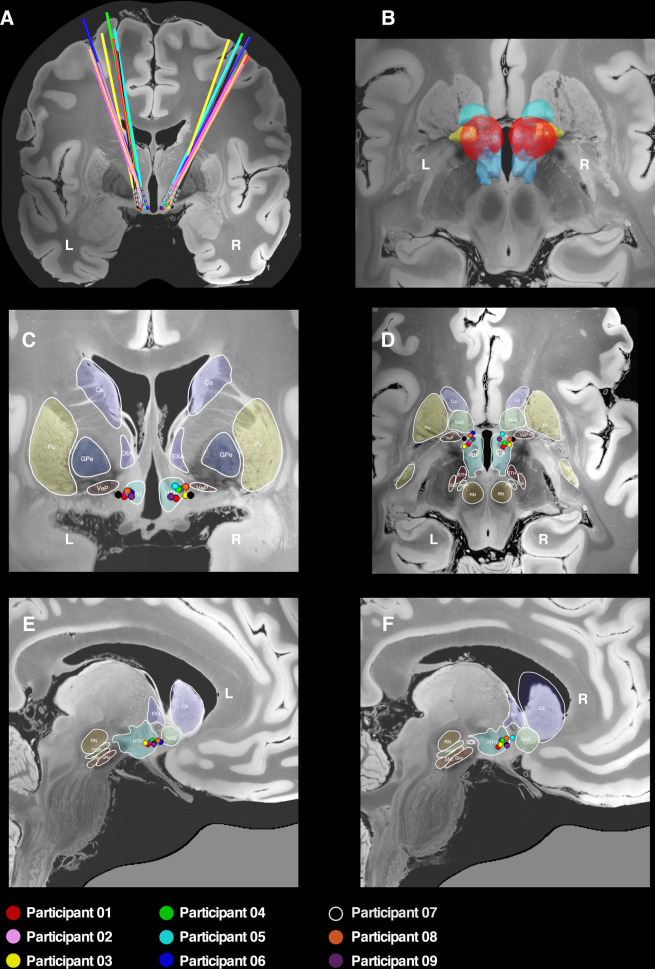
Localisation of electrodes and active contacts. DBS electrodes were localised with the Lead-DBS toolbox and represented in common ICBM 2009b nonlinear asymmetric space incorporating a 7-Tesla MRI at 100 micron resolution^73^ with subcortical parcellations derived from a recent high-resolution atlas^74^. A Three-dimensional reconstruction in the coronal plane showing electrode trajectories for the nine participants. B Three-dimensional reconstruction in the axial plane showing the distribution of the aggregated stimulation field across the cohort (red), which can be seen to encompass the posterior segment of the nucleus accumbens (light green), the ventral pallidum (yellow) and the hypothalamus (blue). C Two-dimensional reconstruction of active contacts in coronal plane. D Two-dimensional reconstruction of active contacts in axial plane. E, F Two-dimensional reconstruction of active contacts in sagittal plane. In the two-dimensional representations, coloured circles represent the second most inferior contact on each electrode (i.e. contact 9 on right electrode and contact 1 on left electrode). Ca caudate, EXA extended amygdala (BNST), GPe globus pallidus external segment, HTH hypothalamus, NAC nucleus accumbens, PBP parabrachial pigmented nucleus, Pu putamen, SN substantia nigra, STH subthalamic nucleus, RN red nucleus, VeP ventral pallidum.
